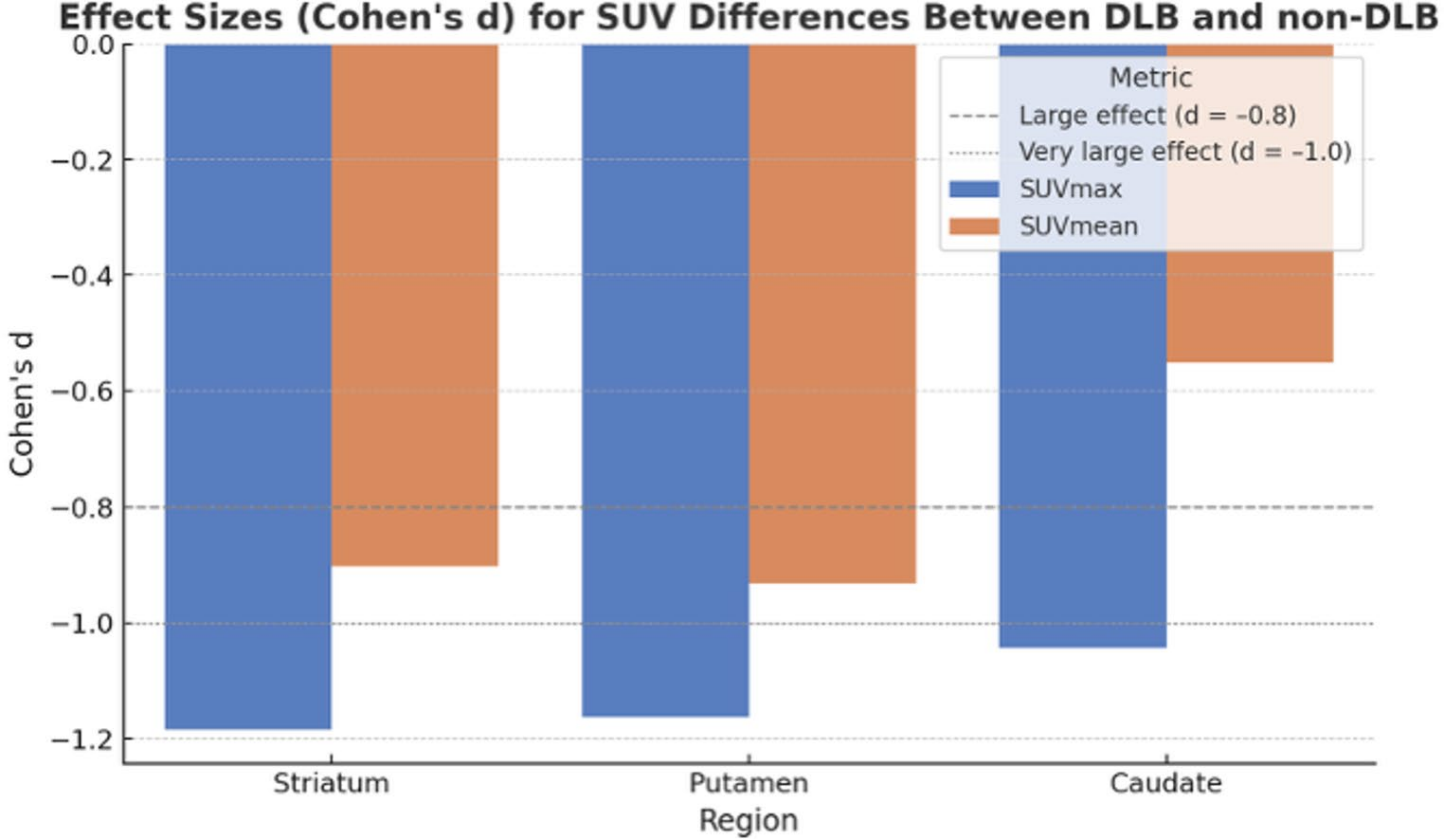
Cohen’s d effect sizes for SUVmax and SUVmean in the striatum, putamen, and caudate nucleus. All metrics show large effect sizes (d < − 0.8), indicating strong dopaminergic differences between DLB and non-DLB patients. Dashed and dotted lines mark the conventional thresholds for large (–0.8) and very large (–1.0) effects
